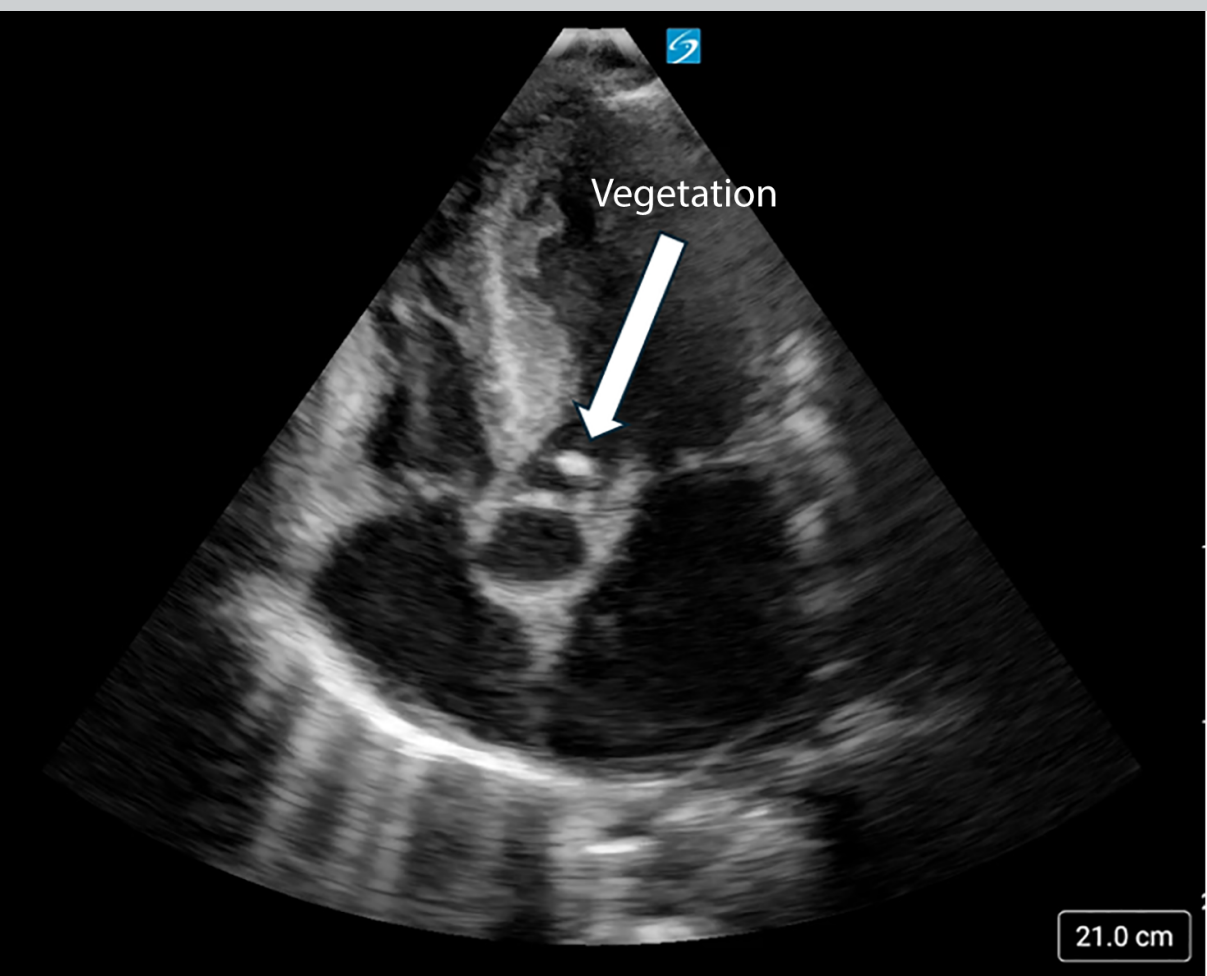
Advanced cardiac point of care ultrasound (POCUS), apical 5-chamber view with a vegetation on the ventricular side of the aortic valve.
